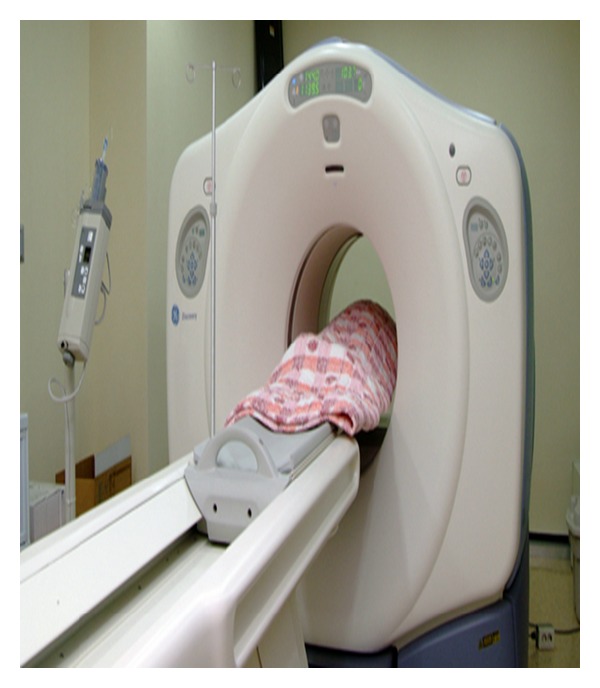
PET/CT scanner (Discovery VCT, GE Milwaukee, USA).
